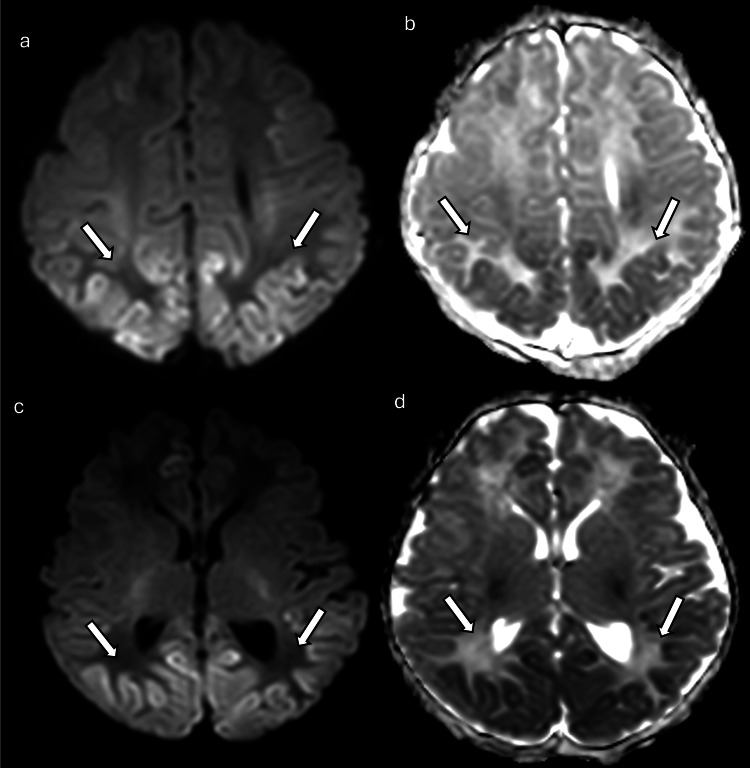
Hypoglycemic encephalopathy in a one-day-old newborn with seizures, lethargy, decreased intake, and low blood sugar (a) and (c) DWI and (b) and (d) ADC maps show areas of restricted diffusion involving bilateral occipital lobes, bilateral posterior temporal lobes, the superior aspect of the vermis of the cerebellum, the dorsal lateral aspect of the midbrain bilaterally, bilateral thalami, bilateral hippocampi, the right side of the cingulate gyrus, and the cortex/subcortical region of bilateral frontotemporal regions (arrows). Images obtained by the authors from the institutional PACS. All images are de-identified and used in accordance with institutional policy. DWI: diffusion-weighted imaging; ADC: apparent diffusion coefficient; PACS: picture archiving and communication system
